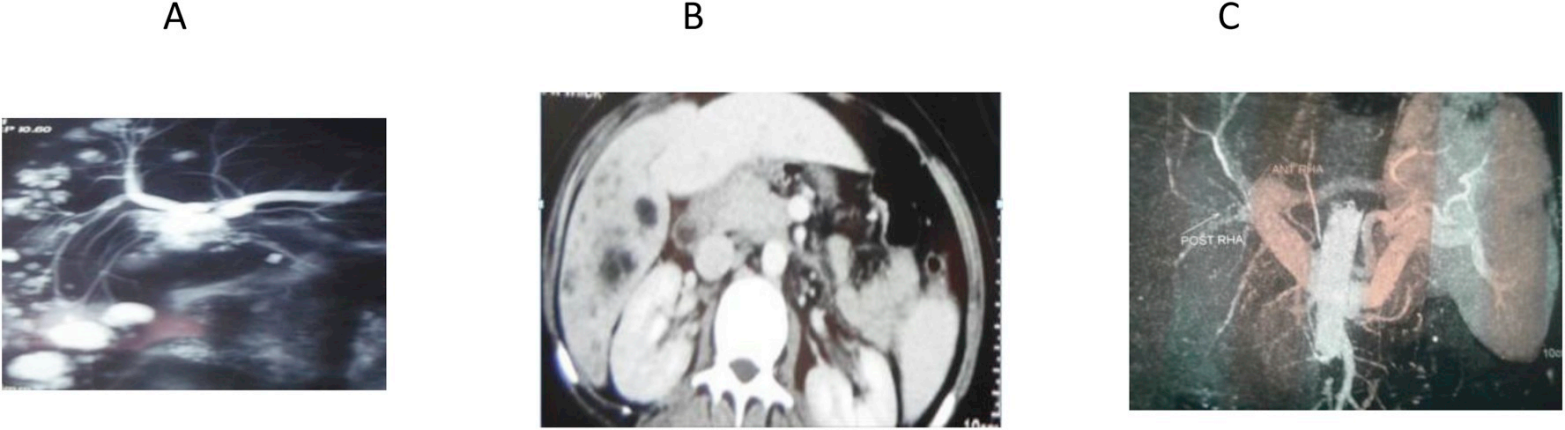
A,B,C: MRCP, CT and CT angiography respectively showing BI type E4 with RT HA and PV injuries and multiple hepatic abscesses in RT lobe, this patient underwent RT hepatectomy with biliary reconstruction.
